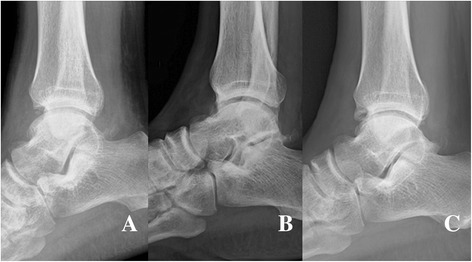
a This plain radiograph obtained at 8 weeks postoperatively did not show the Hawkins sign in the reimplanted talus. b After 24 months, the anterior osteophyte appeared without narrowing at the ankle joint surface. c At the last follow-up at 6 years, the reimplanted talus was well positioned in the ankle joint without avascular necrosis
